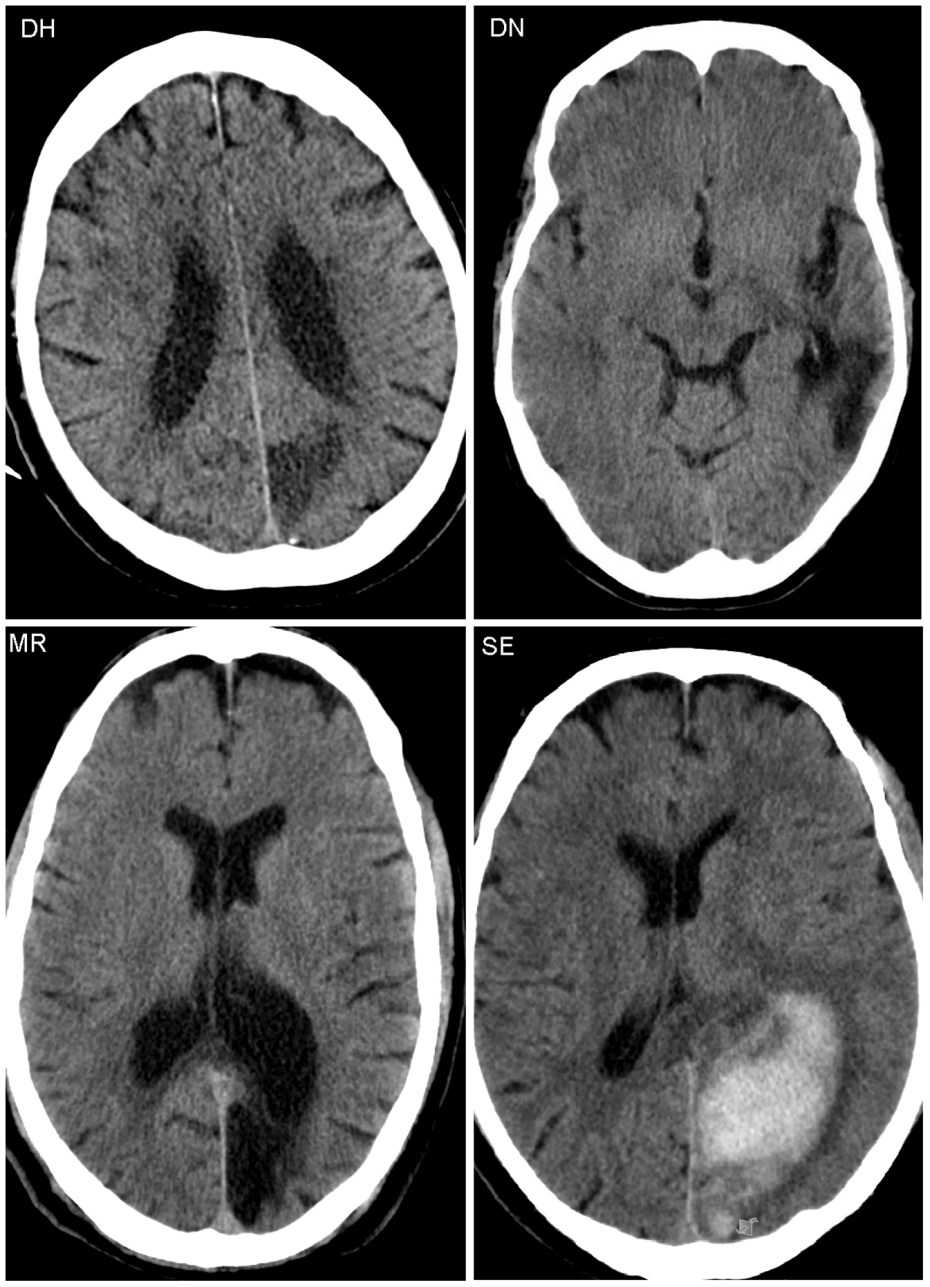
CT scans of the alexic participants.
